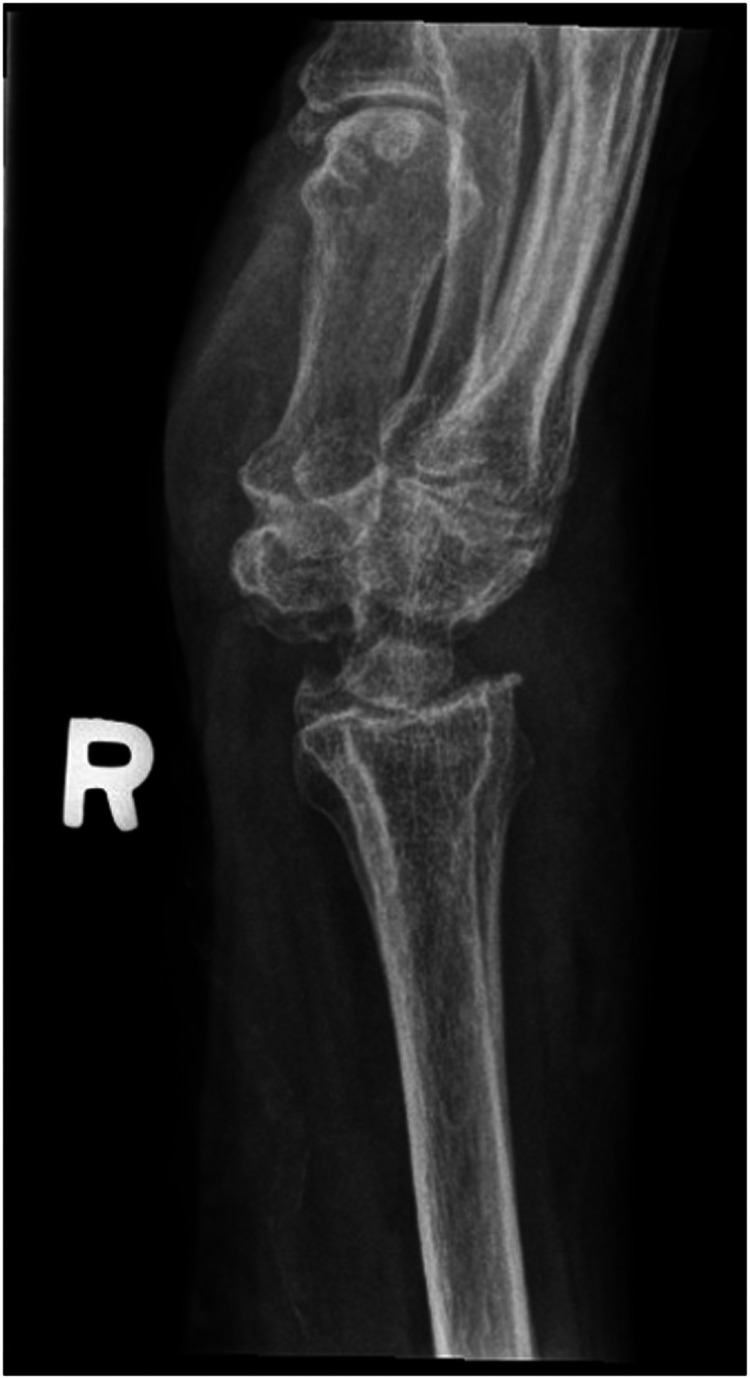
Postoperative right hand plain film lateral view x-ray.
